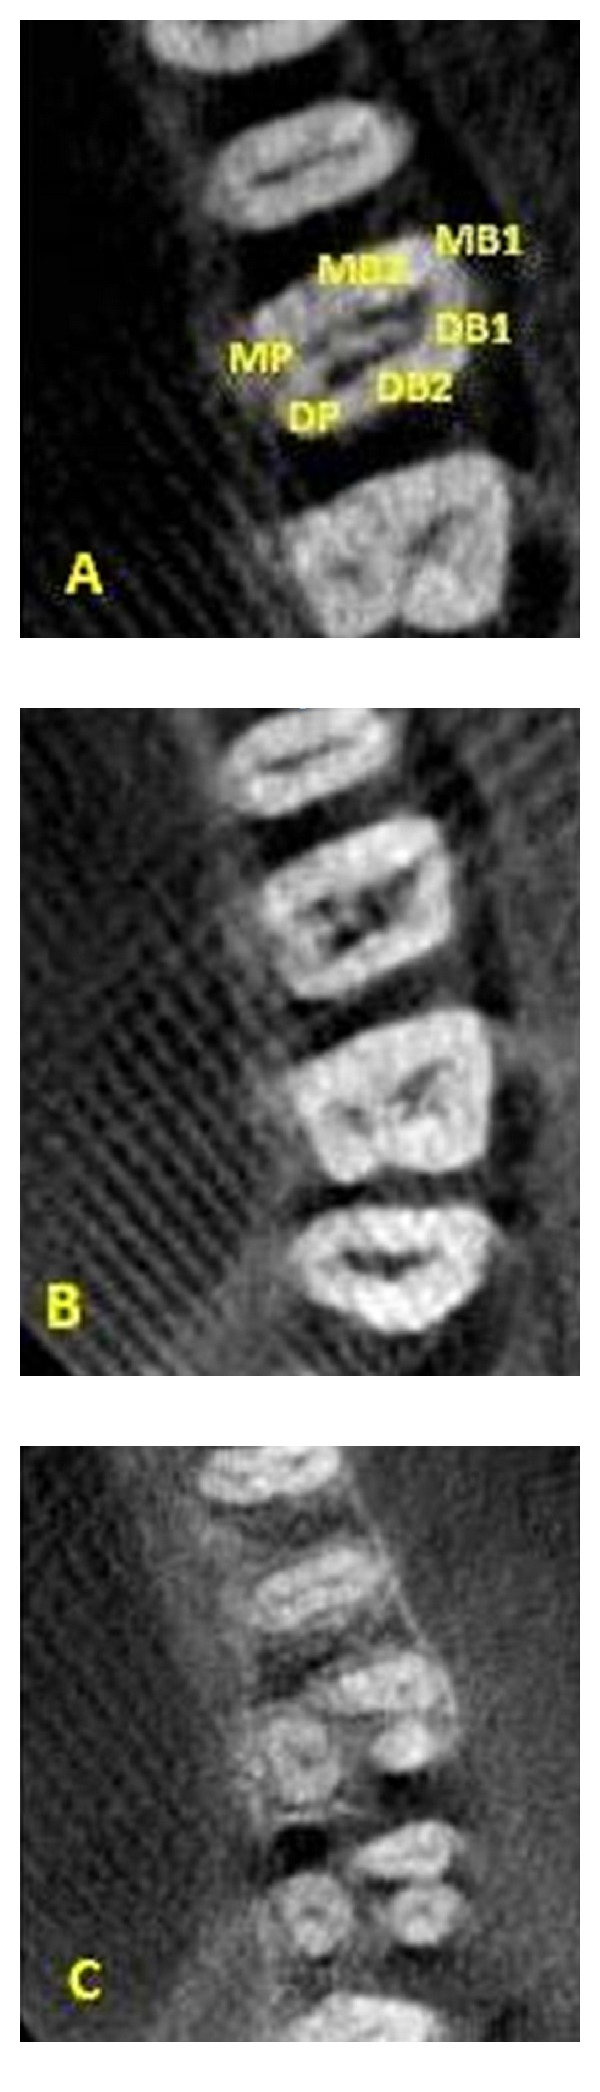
CBCT images showing 2 canals in each root.
